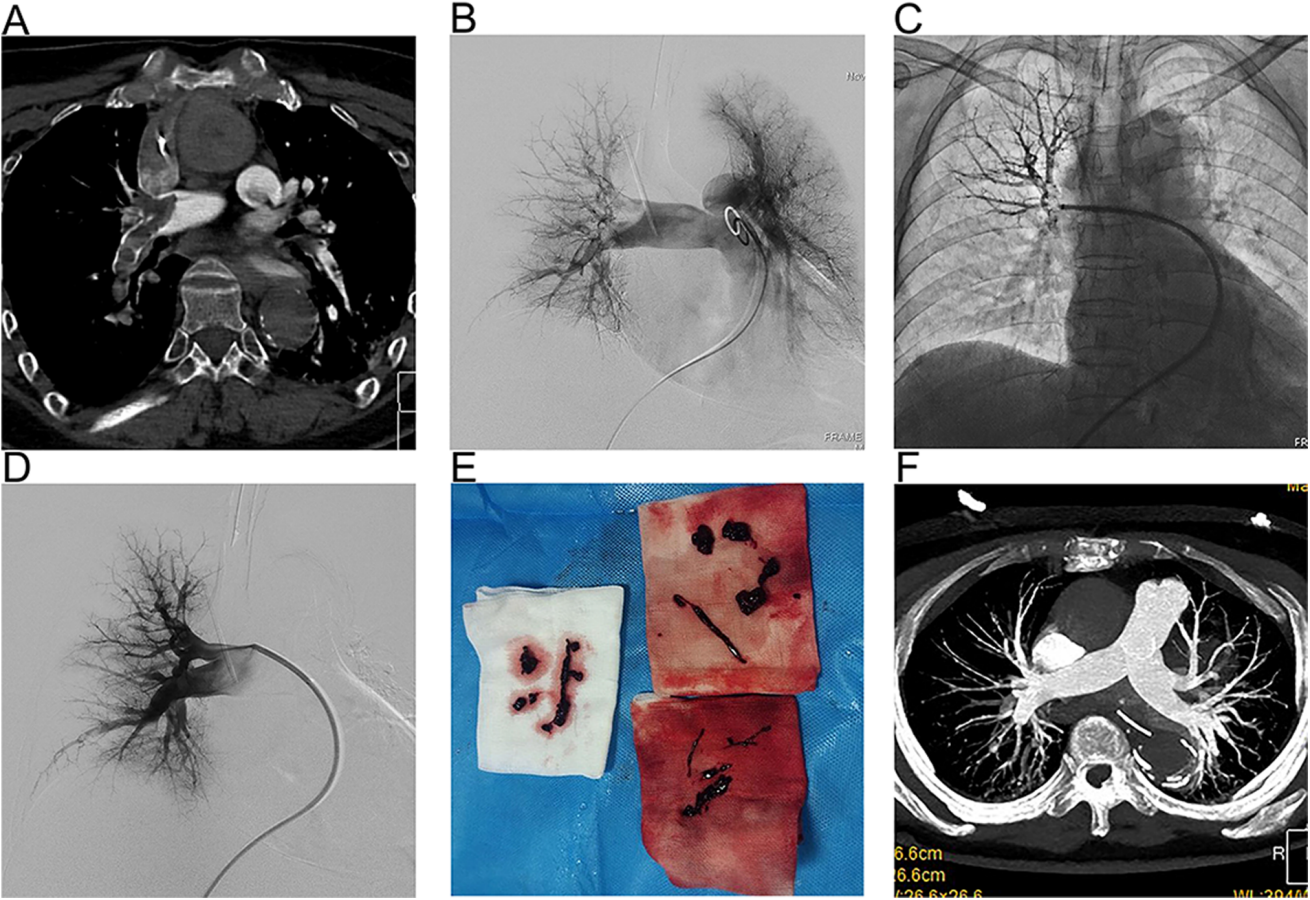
A representative case of VA-ECOM-assisted PMT for PE. (A) CTA revealed a large pulmonary embolism and occlusion of the pulmonary artery; (B) Digital subtraction pulmonary angiograms before thrombectomy shown significant right pulmonary artery obstruction; (C) Application of bolting system; (D)) Angiograms after thrombectomy demonstrated pulmonary artery markedly improved perfusion; (E) The thrombus was obtained during thrombectomy; (F) Follow-up CTA demonstrating no thrombus in the pulmonary artery.
